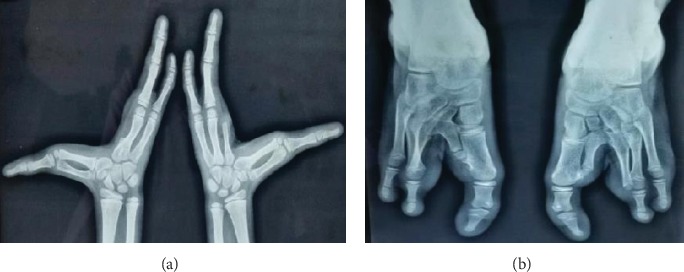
(a) Radiograph of a hand showing syndadactyly of 1^st^ and 2^nd^ metacarpals with aplasia of 2^nd^ and hypoplasia of 3^rd^ phalange. (b) Radiograph of a foot showing syndactyly of 1^st^ and 2^nd^ metatarsals with aplasia of 2^nd^ and hypoplasia of 3^rd^ phalanges.
